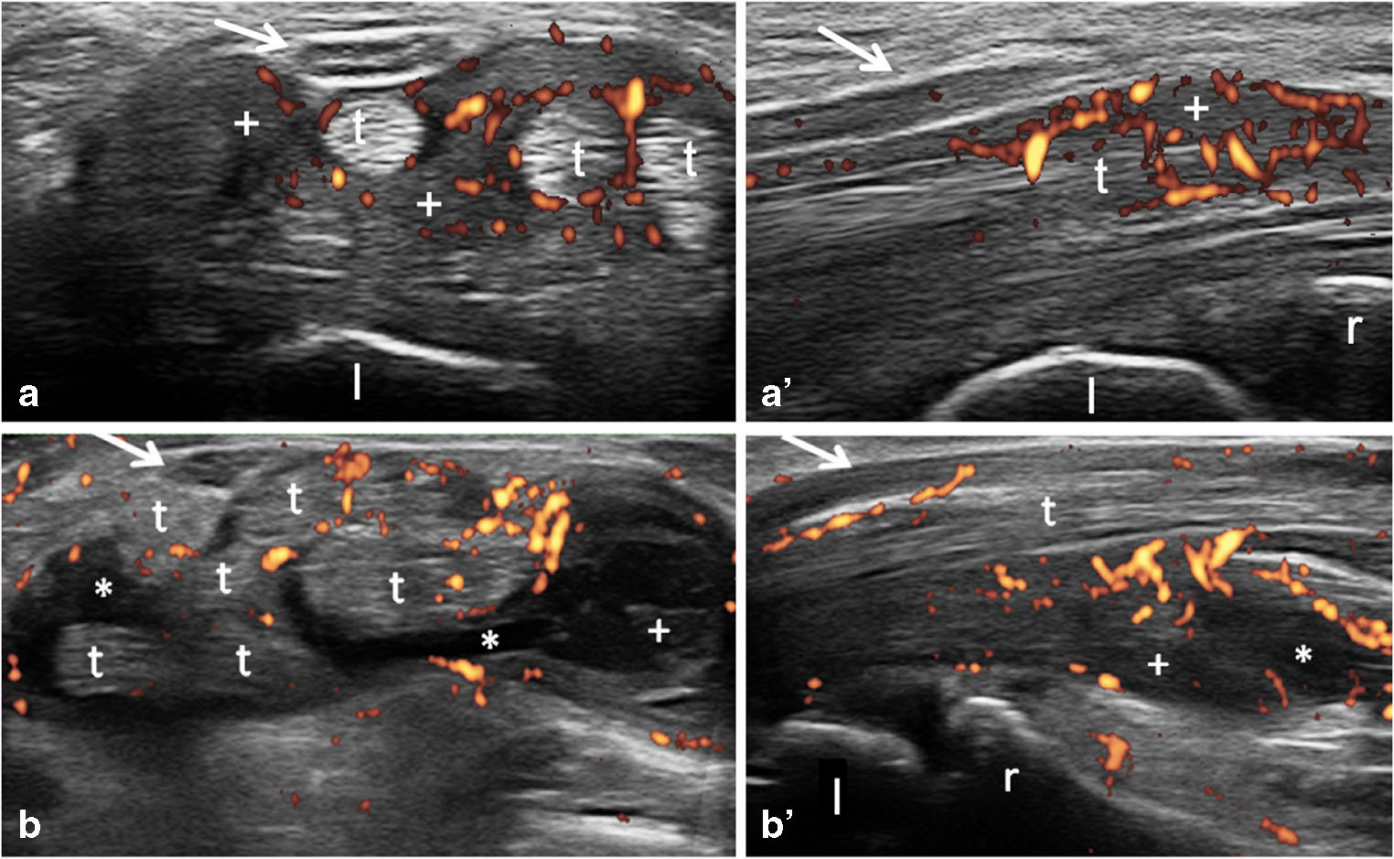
Rheumatoid arthritis. Carpal tunnel syndrome. Representative ultrasound images obtained in transverse (a and b) and longitudinal (a′ and b′) scans showing tenosynovitis of finger flexor tendons. Note the abnormal tendon sheath widening due to inflamed areas of synovial hypertrophy (plus signs) with evident power Doppler signal and effusion (asterisks) and no relevant morphostructural changes of the median nerve (arrows). The image in b was acquired slightly proximally to carpal tunnel inlet in order to better depict the inflammatory abnormalities. r = radius, l = lunate bone, t *=* finger flexor tendons
